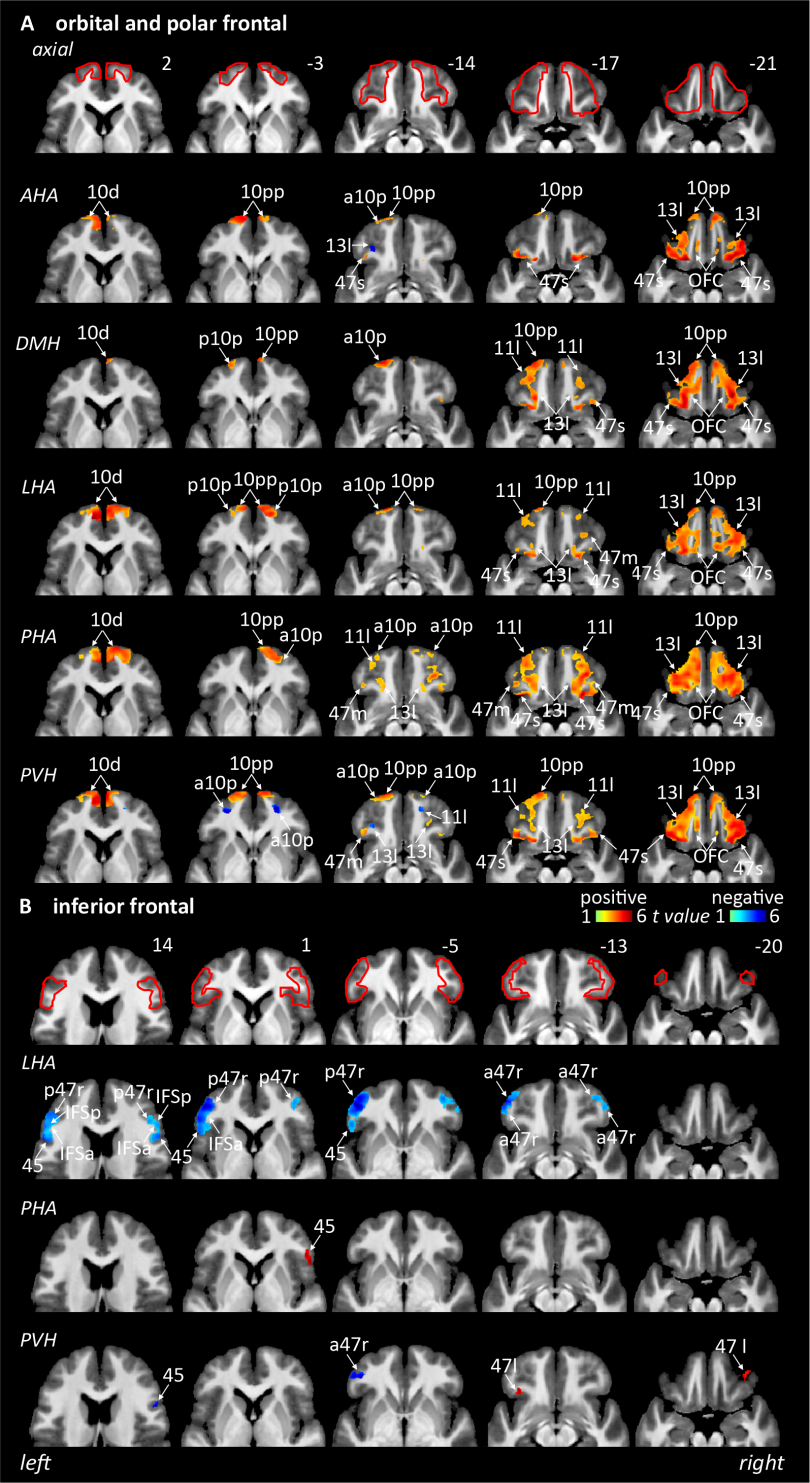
Resting connectivity between six hypothalamic seed regions and the orbital/polar frontal and inferior frontal cortical regions (n = 150). Areas of significant positive (hot colour scale) and negative (cool colour scale) connectivity overlaid onto a series of axial mean T1-weighted anatomical images. Slice locations in Montreal Neurological Institute Space are indicated at the top right of each slice. Red outlines indicating region of interest masked applied for the analysis. (A) Orbital and polar frontal region. (B) Inferior frontal region. For abbreviations, see table.
